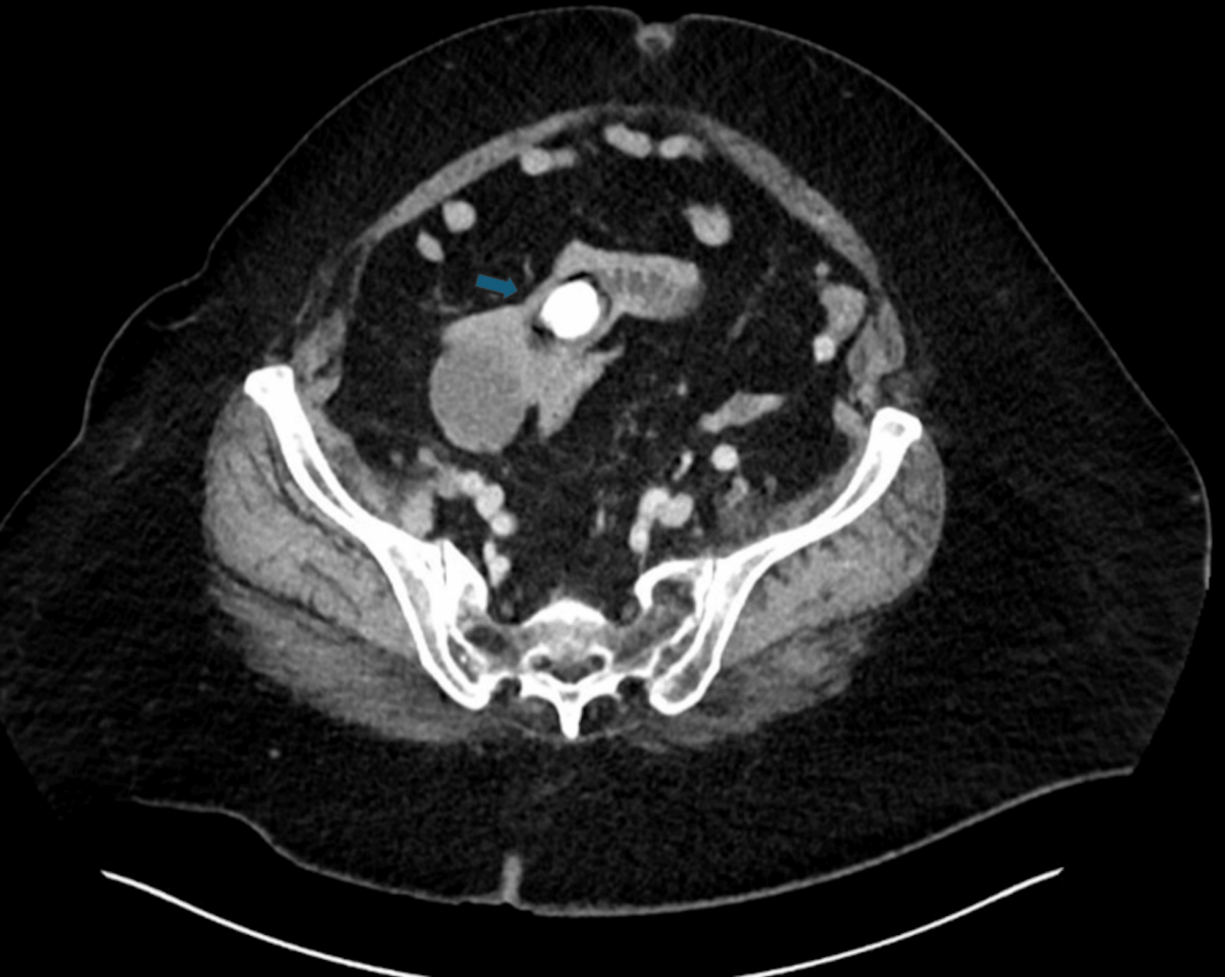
Calcified mass in the small bowel This horizontal CT image of the abdomen demonstrates a 2 cm calcified mass in the distal small bowel (blue arrow).
